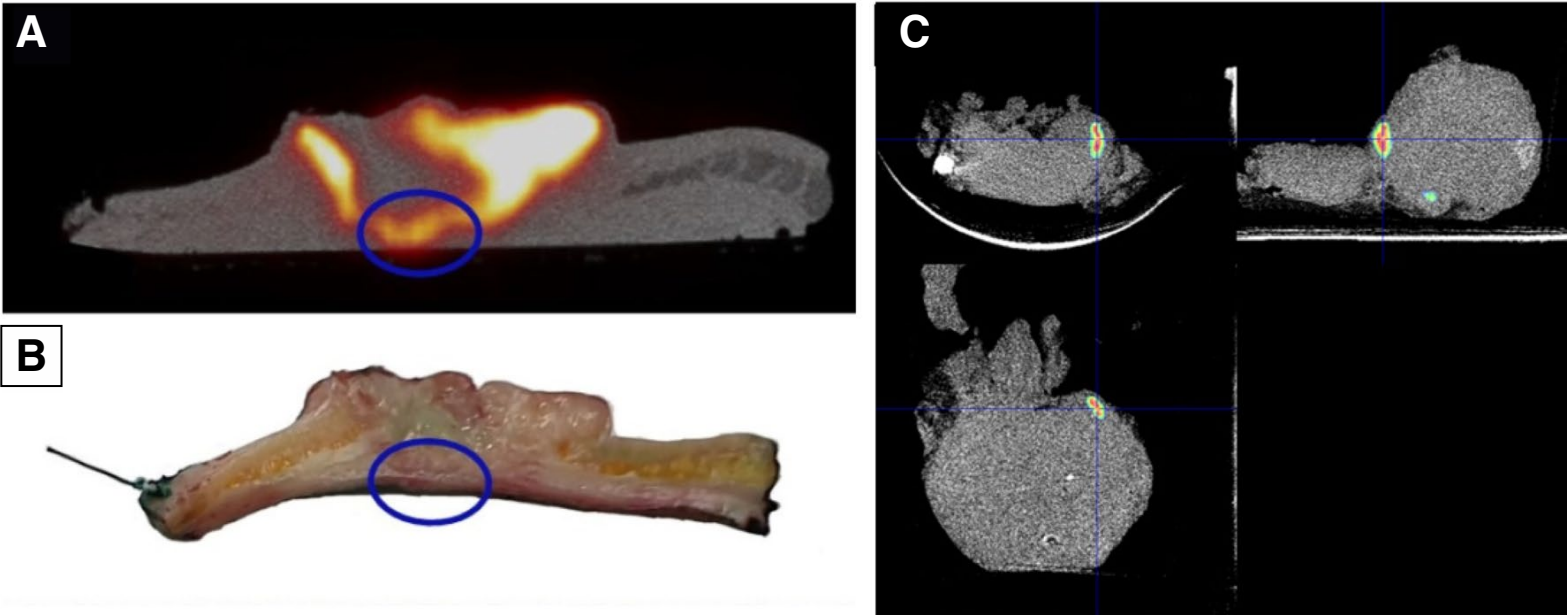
PET/CT imaging in the assessment of intraoperative margins*.* Back table PET/CT imaging, after 2-[^18^F]FDG administration, of a cutaneous squamous cell carcinoma (A) and the sliced tissue of the proposed region corresponding with the PET/CT imaging (B) [86]. PET/CT imaging of the prostate preparation after preoperative [^18^F]PSMA-1007 injection with evidence of tracer-uptake immediately at the resection margin (C) [84]
